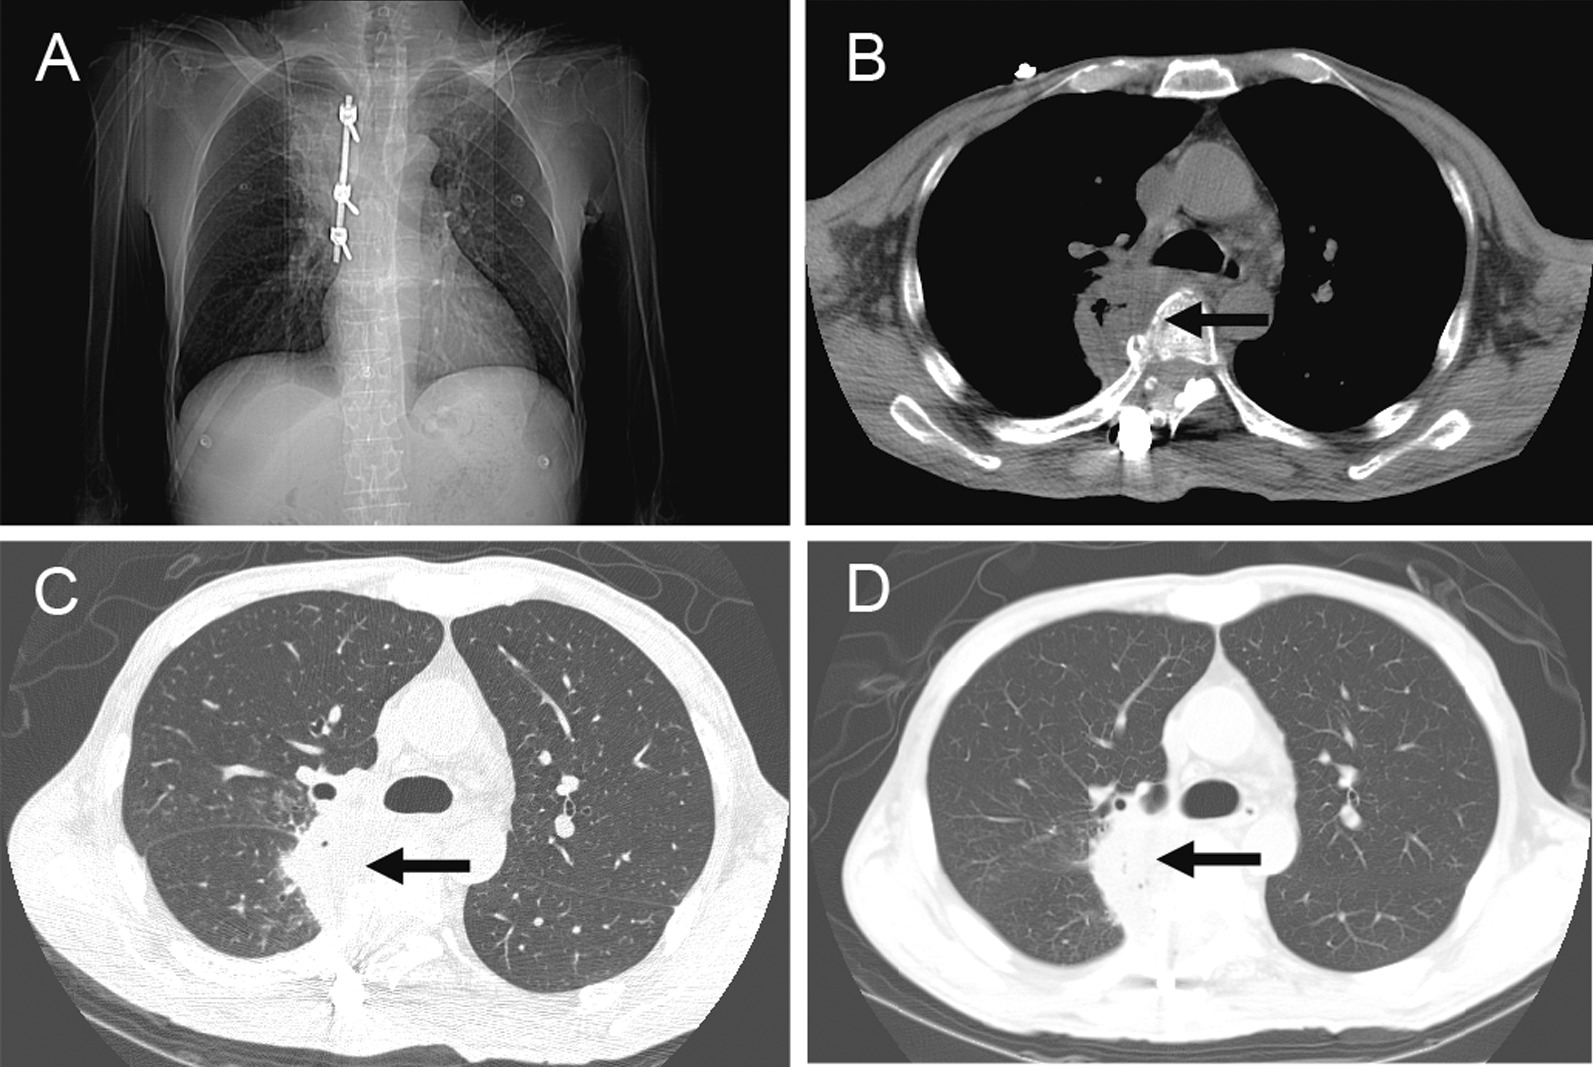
The right lung abscess still existed, but the pneumonia was alleviated (black arrow). A Chest CT images were reviewed 5 days after the operation. B Chest CT images were reviewed 5 days after the operation. The abscess cavity decreased and pleural effusion disappeared. C and D Reexamination at 16 and 21 days after surgery showed no significant changes in lung inflammation
